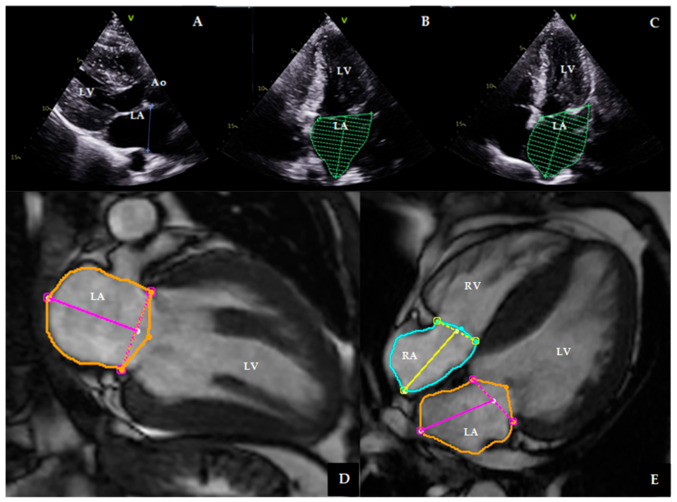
(A) LA anteroposterior diameter measurement by TTE. (B) Two−chamber and (C) 4−chamber LA volume measurement by TTE. (D) Two−chamber and (E) 4−chamber LA and RA volume measurement by CMR. The orange lines represent the LA perimeter, the pink lines represent the maximal LA diameter, the blue line represents the RA perimeter, and the yellow line represents the maximal RA diameter. Ao: Aorta; CMR: Cardiac magnetic resonance; LA: Left atrium; LV: Left ventricle; RA: Right atrium; RV: Right ventricle; TTE: Transthoracic echocardiography.
